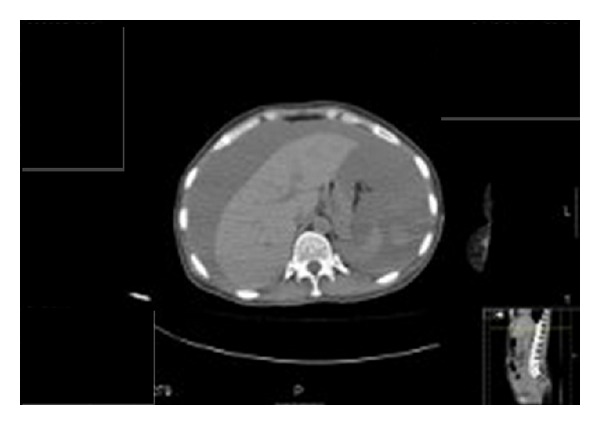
CT scan demonstrating significant intraperitoneal bleeding.
